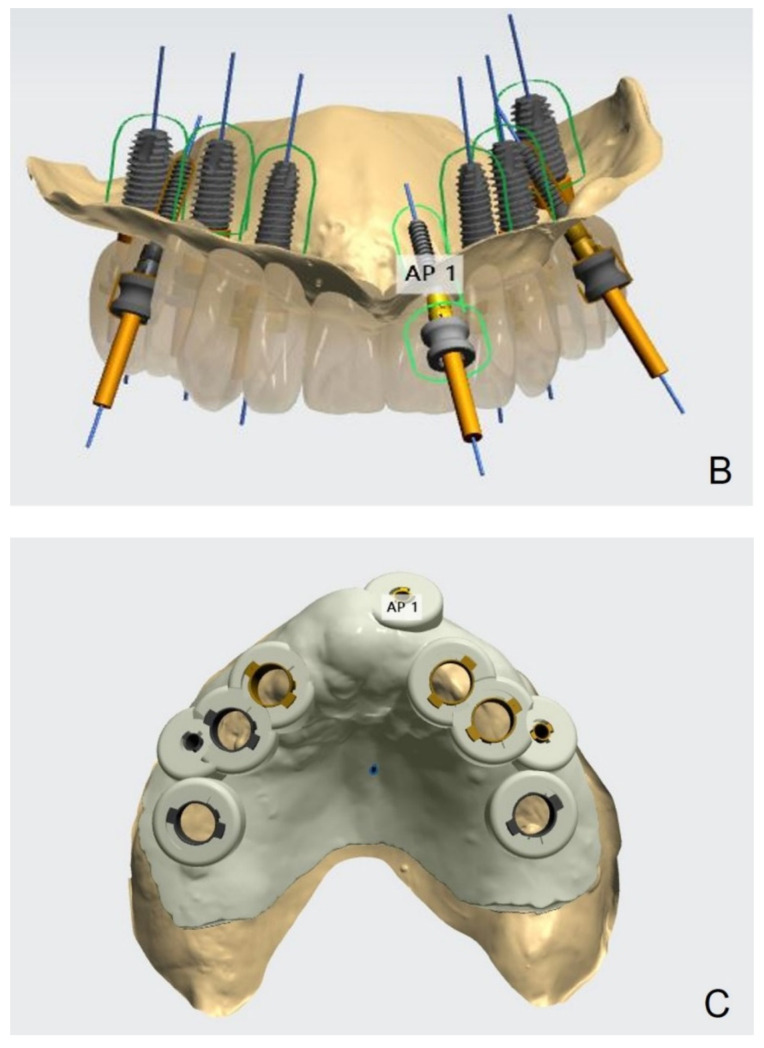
(A) Image fusion by matching resin markers present on the intraoral scan and CBCT. (B) Virtual implant planning. (C) Designed guide for the placement of six dental implants.
